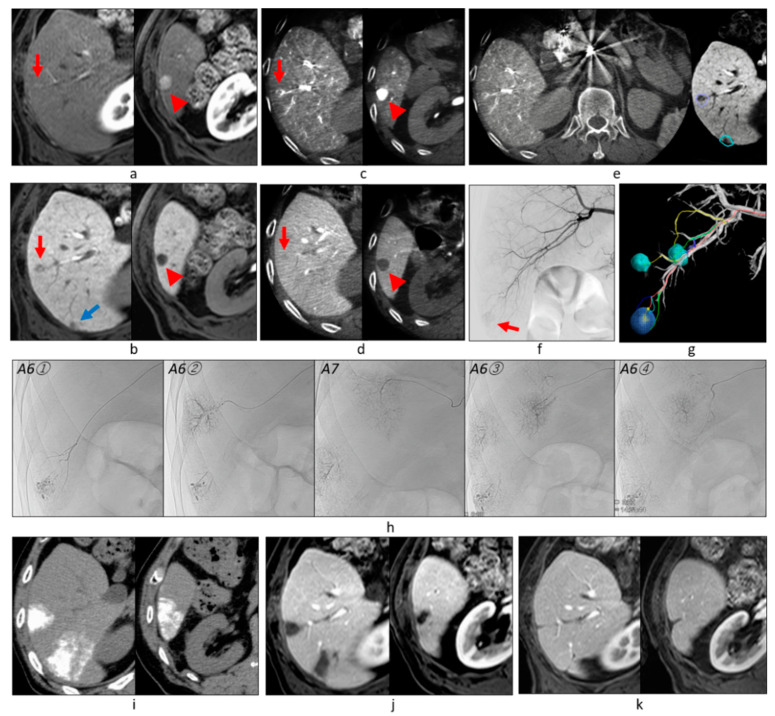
Overlay image fusion technique. (a) Arterial-phase images of gadoxetate disodium-enhanced MRI showed overt HCC (arrowhead) and well-differentiated HCC (red arrow). (b) Hepatobiliary-phase images also depicted early HCC (blue arrow). CBCTAP (c) and first-phase CBCTHA (d) showed overt HCC (arrowhead) and well-differentiated HCC (red arrow), but early HCC could not be identified. (e) Thus, early HCC was segmented after overlay image fusion between the first-phase CBCTHA and MRI images. (f) Common hepatic arteriogram showed only one tumor (arrow). (g) On the other hand, AFD could identify all tumor feeders. (h) Then, all tumor feeders were embolized without performing selective DSA of each feeder. (i) Iodized oil was densely accumulated in the highly limited area, including the tumors on unenhanced CT performed 1 week after TACE. (j) MRI performed 2 months after TACE showed that the embolized areas were necrotized regardless of tumor vascularity. (k) The tumors have remained well controlled for 4 years. Figure 2a–d,f–j are reprinted with permission from [24].
